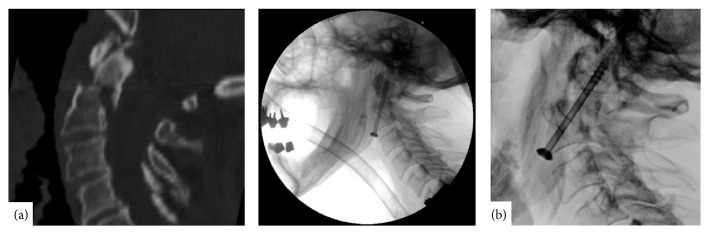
(a) Preoperative computed tomography (CT) of the odontoid fracture, sagittal view (left). Intraoperative view on odontoid screw placement, lateral view (right). (b) X-ray imaging disclosed a five-millimeter dorsal migration of the odontoid process three weeks after the operation, lateral view.
